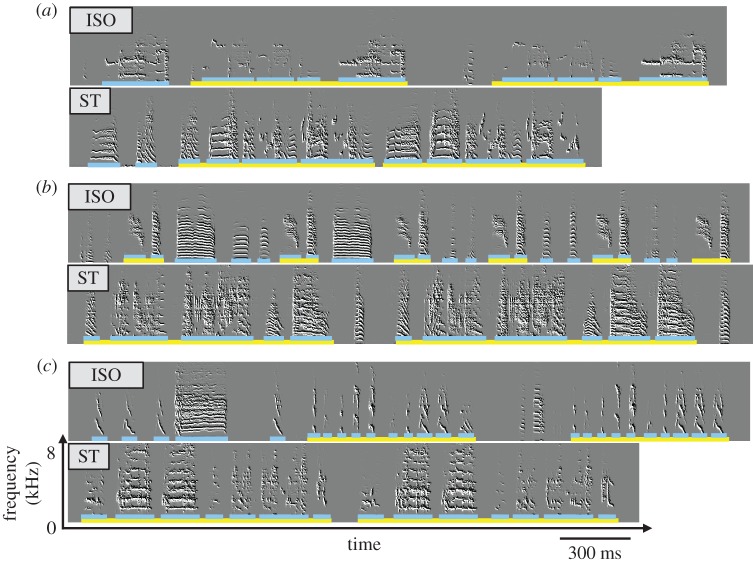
Adult songs of ISO and ST (ST) sibling pairs. Sonograms of three pairs are shown (*a–c*). Songs of the ISO brothers are above their ST siblings'. Song motifs are underlined in yellow, song syllables in blue. ST motifs are more stable (repeated in the same way every time), composed of more syllable types and are generally longer. ST syllable durations are similar to the WT durations; ISO durations tend to be too short or too long.
